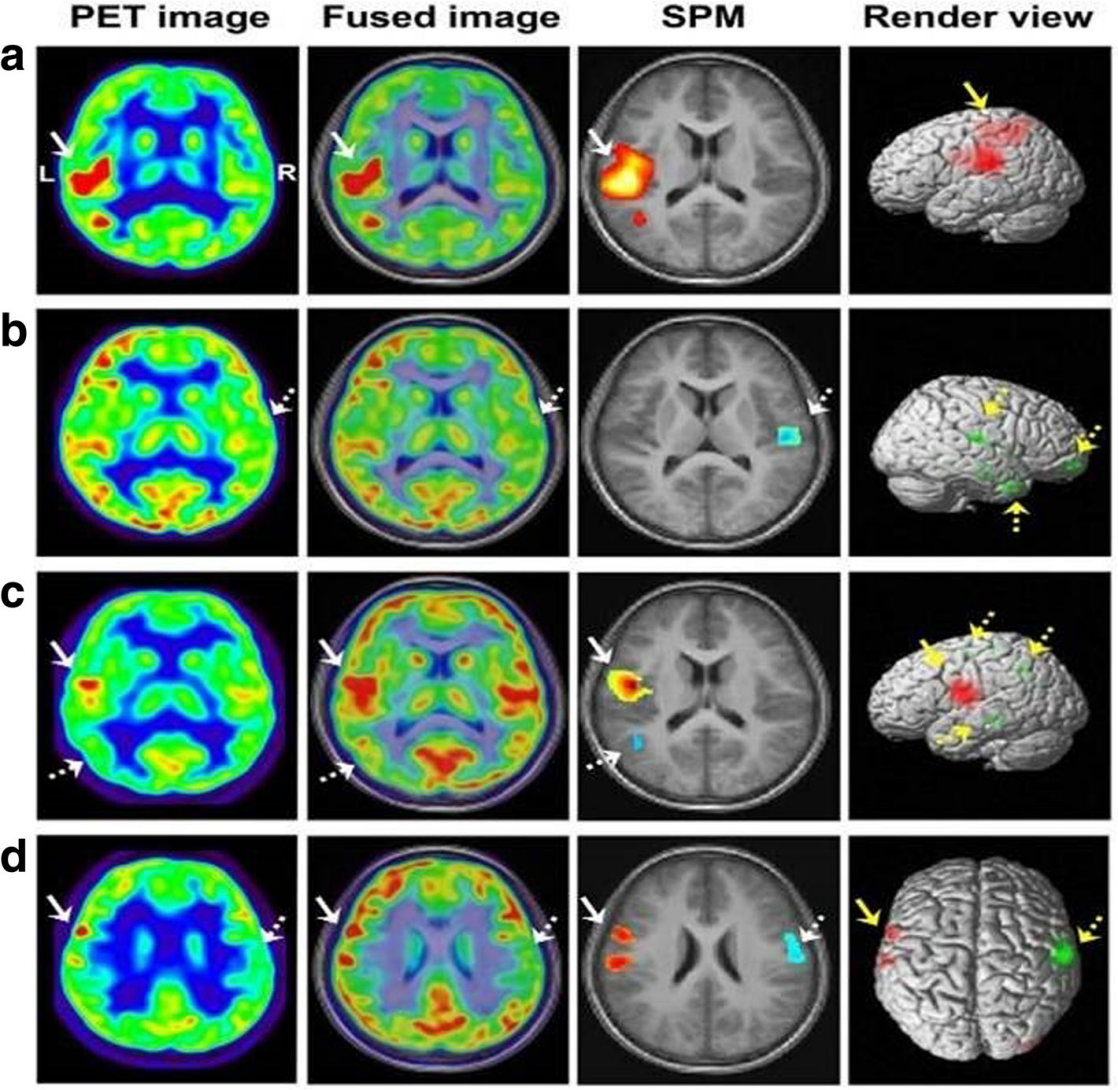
Comparison between visual and SPM analysis of 18F-FDG PET images. (a) Hypermetabolism in bilateral frontal lobes (solid arrow) was detected by both visual and SPM analysis. (b) Hypometabolism in left temporal lobe (solid arrow) was detected by both visual and SPM analysis. (c) Hypometabolism was found in left rolandic area (dashed arrow) by visual assessment, and hypermetabolic region was further identified in right rolandic area (solid arrow) by SPM analysis. (d) Hypometabolic region undetected by visual assessment was identified in left mesial temporal lobe (solid arrow) by SPM analysis. (This research was originally published in Y. Zhu et al., “Glucose Metabolic Profile by Visual Assessment Combined with Statistical Parametric Mapping Analysis in Pediatric Patients with Epilepsy, (Zhu et al. 2017)” J. Nucl. Med., vol. 58, no. 8, pp. 1293–1299, 2017.© by the Society of Nuclear Medicine and Molecular Imaging)
